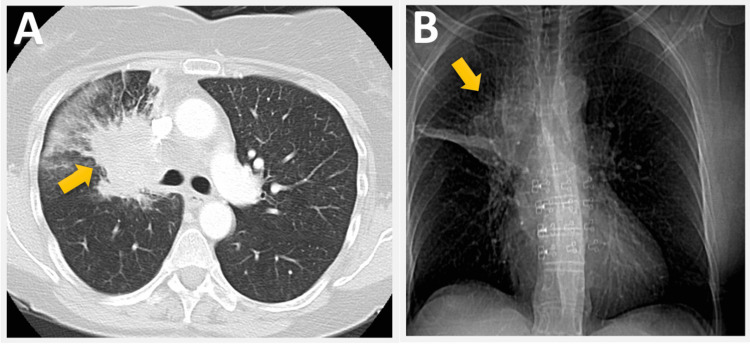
CT scan of the chest demonstrating a large spiculated 7.5 x 6 cm mass in the right upper lobe (arrow in A) and extensive mediastinal lymphadenopathy (arrow in B).
